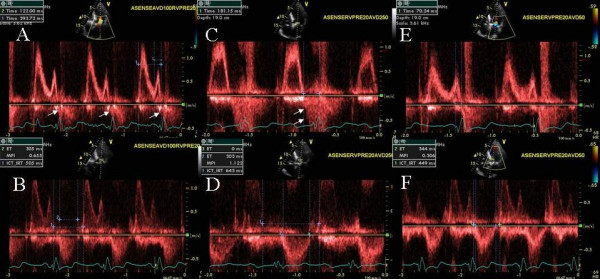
Top panels show the pulsed wave mitral inflow Doppler at AVD of 100, 250 and 50 ms and bottom panels show mitral inflow and aortic outflow PW Doppler at the same settings. Marked E and A fusion and diastolic mitral regurgitation (white arrows) is shown in panel B along with reduced aortic ejection time and marked increase in myocardial performance index as shown in panel D. A marked improvement in mitral diastolic filling time and E and A separation is shown in panels A and E at an AVD of 100 and 50 ms respectively and a corresponding improvement in LV ejection time as well as myocardial performance index as shown in panels B and F. Note that diastolic mitral regurgitation is still present at an AVD as short as 100ms (white arrows panel A).
